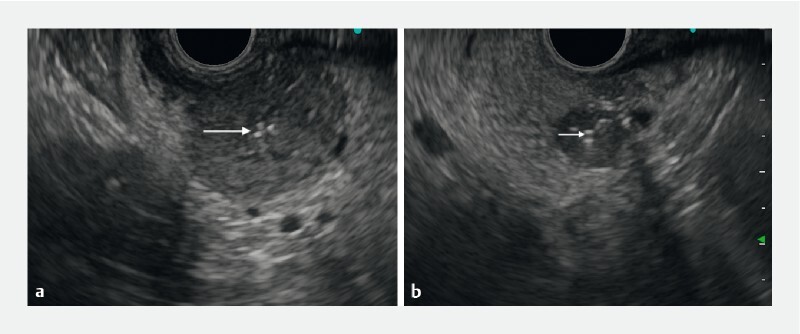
Endoscopic ultrasound (EUS) images showing the fiducial markers, visible as bright echoes (arrows) in the center of pancreatic metastases from renal cell carcinoma, which are seen as hypoechoic tumors in the:
